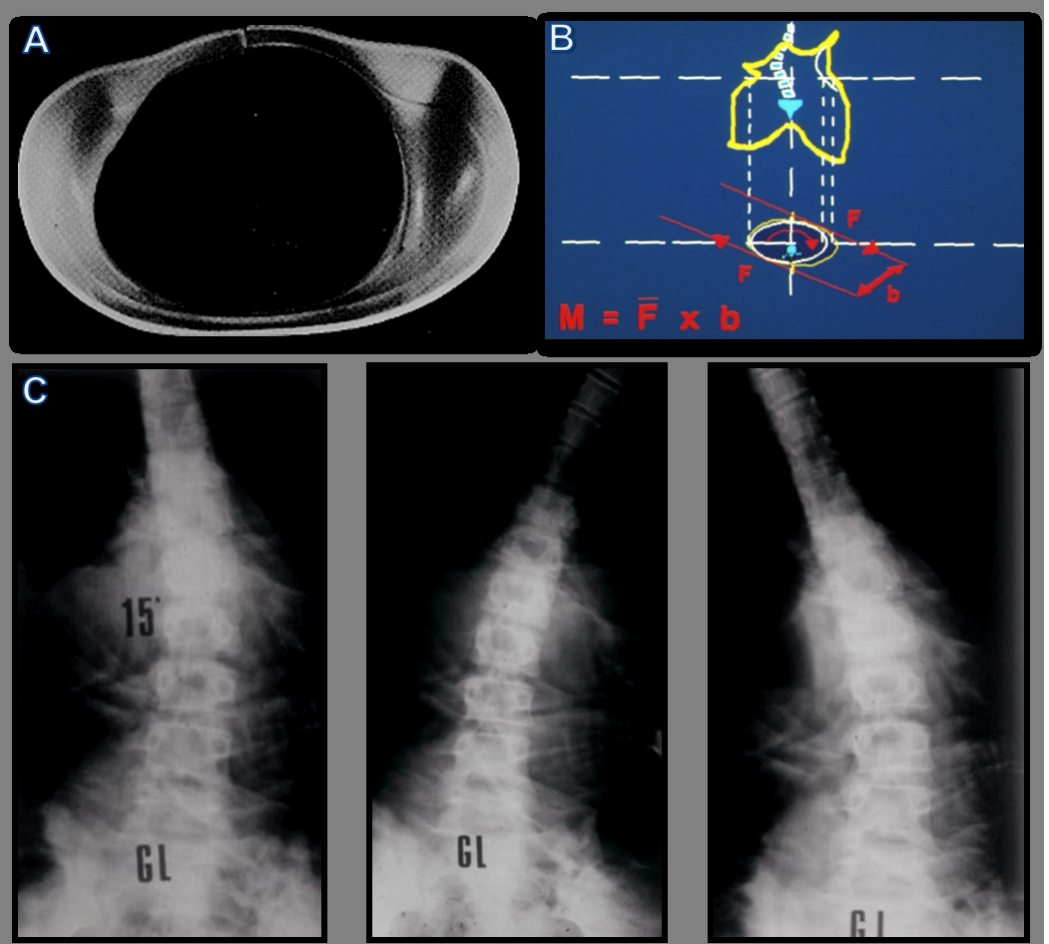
Dynamics bound by the geometry of the brace. The trasverse section of the brace (A), elliptical and asymmetrical up to the plan of the pelvic Hold, produces twisting moments opposed to the direction of the rotation of the vertebrae included in the curve (b). The bending X-ray (c) shows the efficacy of the twisting action produced by the brace for all movements of the trunk.
